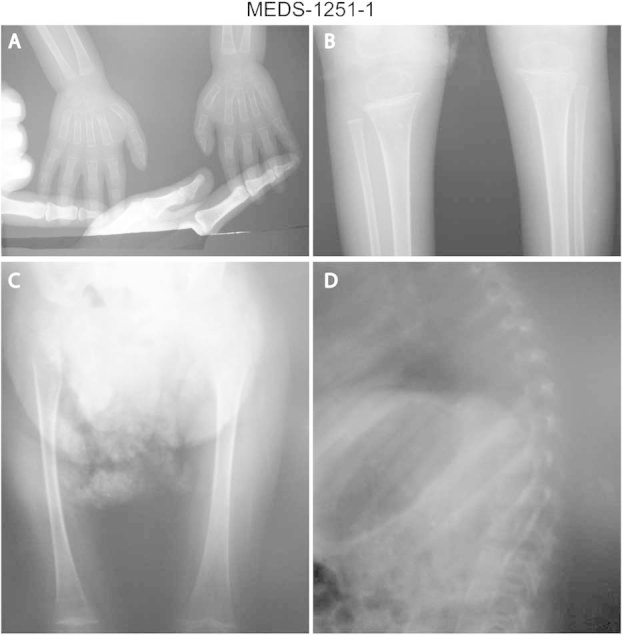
Radiograph of Patient 1. A: Osteopenia in carpal, metacarpal and phalanges with cone shaped proximal phalanges. B: Thin cortex of tibia and fibula with metaphyseal widening and osteopenia. C: Thin cortex of femora with severe osteopenia. D: Kyphosis, severe osteopenia with platyspondyly and osteopenic ribs.
